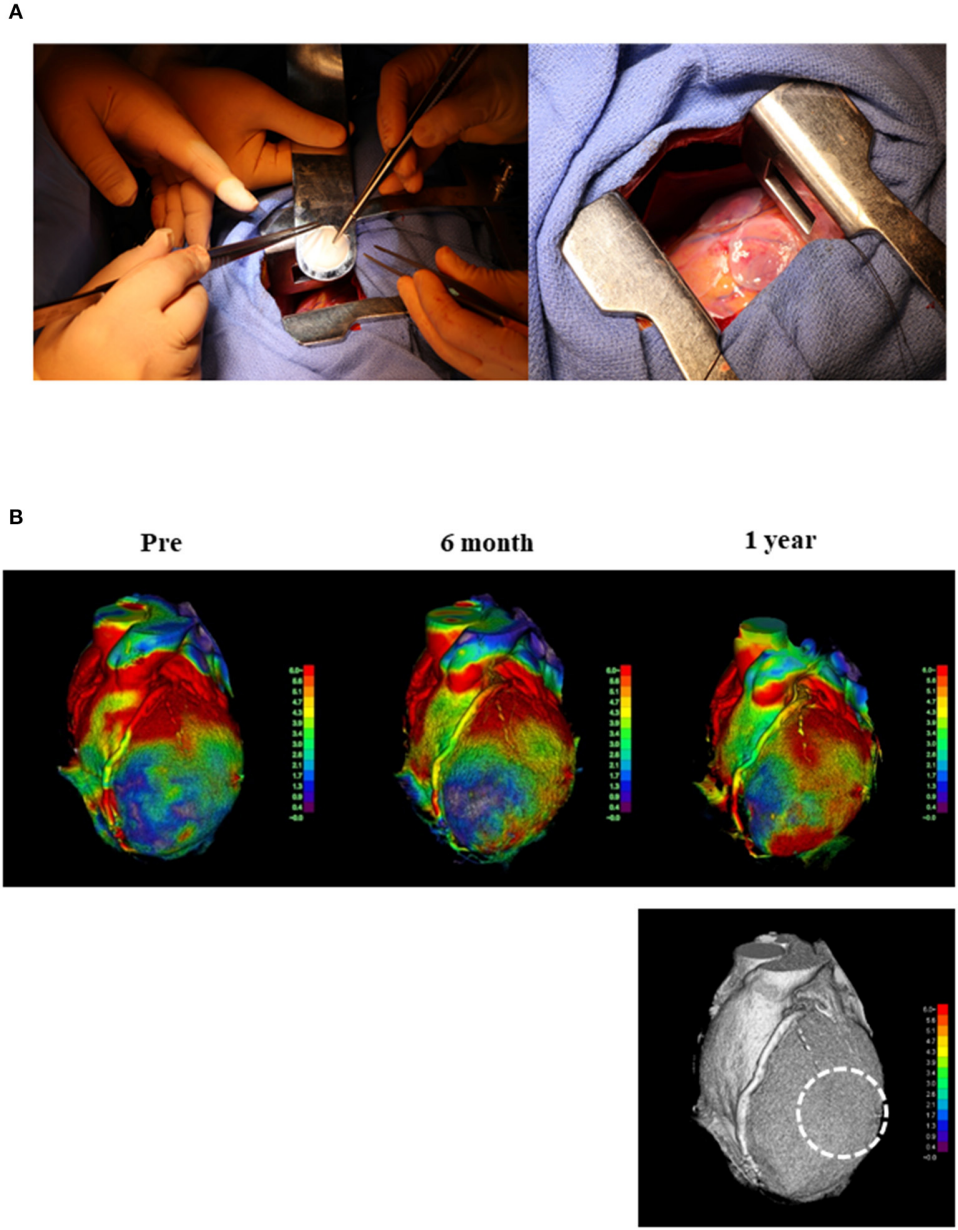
Transplantation of hiPSC-CM and cardiac moving pattern before and after surgery. (A) First transplantation of hiPSC-CM patches onto the heart surface of a patient with severe cardiomyopathy. Left: hiPSC-CM patch was transplanted into the epicardium of the anterior and lateral walls of the LV through the fourth intercostal space under IABP support. Right: Three patches were transplanted into the epicardium of the LV anterior and lateral walls. (B) The moving pattern observed via four-dimensional CT. Colors were set so that red indicated a good dynamic area; the darker the color, the lower the movement. The images show the moving pattern in chronological order of the heart at pre-implantation (pre), 6 months, and 1 year after implantation. The dotted line in the bottom figure shows the area where the patches were attached. hiPSC-CM, human induced pluripotent stem cell-derived cardiomyocyte; LV, left ventricle; IABP, intra-aortic balloon pumping; CT, computed tomography.
